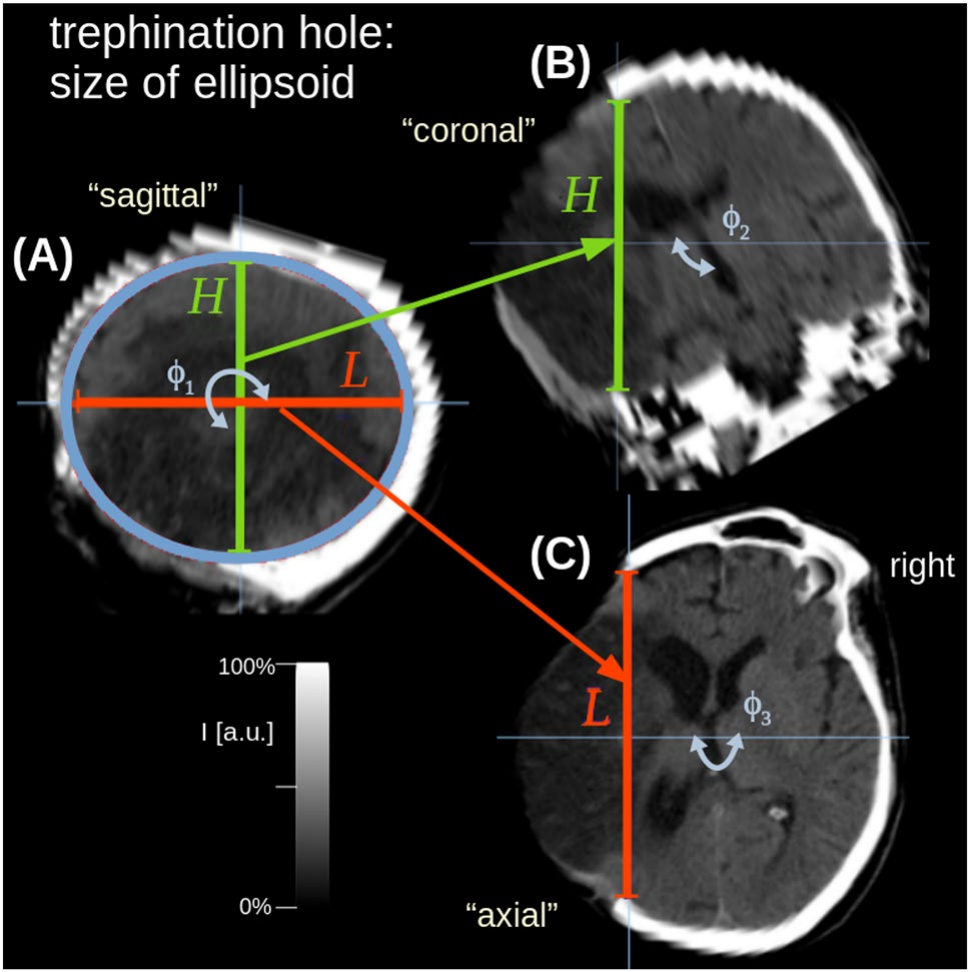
Non-contrast computed tomography CT scan in representative slices after alignment (rotation angles ϕ_1_, ϕ_2_, ϕ_3_) of the trephination hole: A “sagittal” slice aligned with the trephination hole as an ellipse for calculation of the surface area (light blue); B “coronal” slice with the height of the minor axis (H, green); C “axial” slice with the major axis defining the “diameter” of the hemicraniectomy (L, red)
